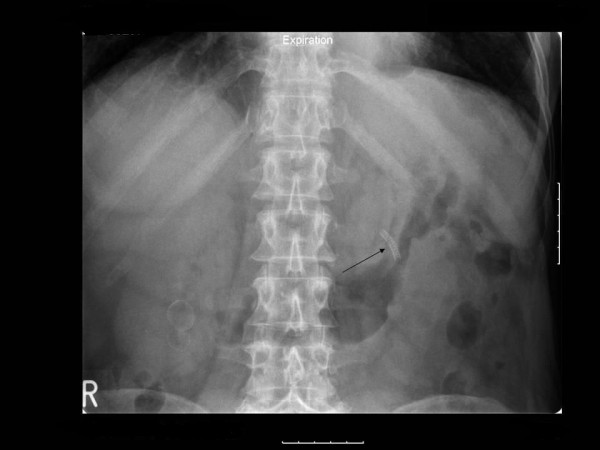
A plain abdominal X-ray film. Six weeks postoperatively, a plain abdominal X-ray confirmed stone-free status and the presence of a covered stent graft in the anatomic location corresponding to the left kidney (arrow).
